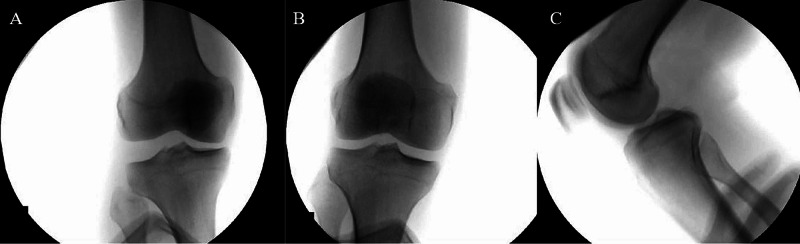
Preoperative right knee stress radiographs. Right knee preoperative stress radiographs demonstrate LCL laxity (A), an intact MCL (B), and excess tibial posterior translation (C). MRI was not available preoperatively due to the left knee external fixation. LCL: lateral collateral ligament; MCL: medial collateral ligament; MRI: magnetic resonance imaging
